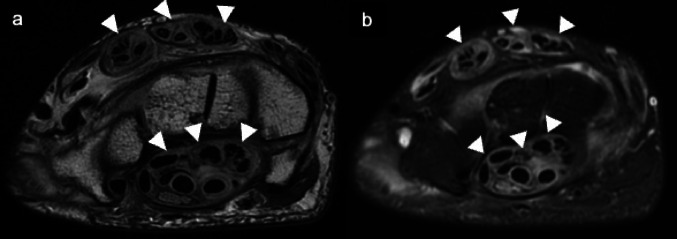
Rheumatoid arthritis. Axial T2-weighted (a) and fat-suppressed T2-weighted MR images (b) show thickened tendon sheaths surrounding the flexor and the extensor tendons (arrowheads)
